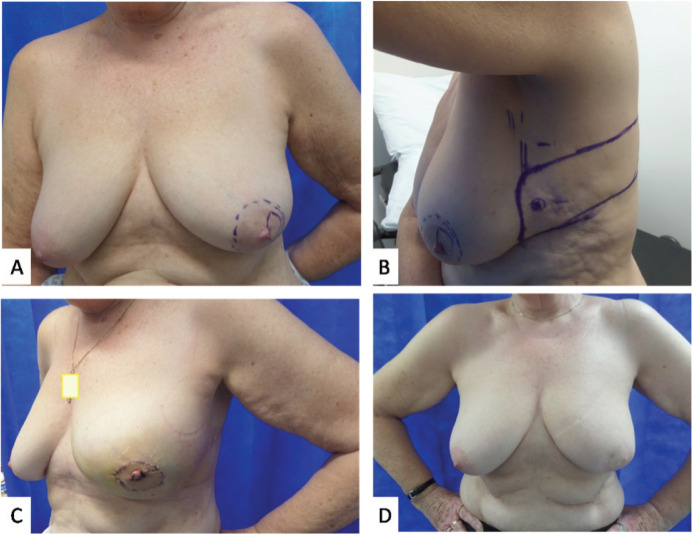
Photographs of case 2. (a): Marked sub-areolar lump with dashed markings for proposed central excision. (b): Lateral view showing the markings of CWPF with cross marked at perforator as identified using a handheld Doppler. (c): 2 weeks post-operative results showing reconstructed central defect with nipple and areolar reconstruction from skin of the flap. (d): 1 year post surgery and post radiotherapy results.
